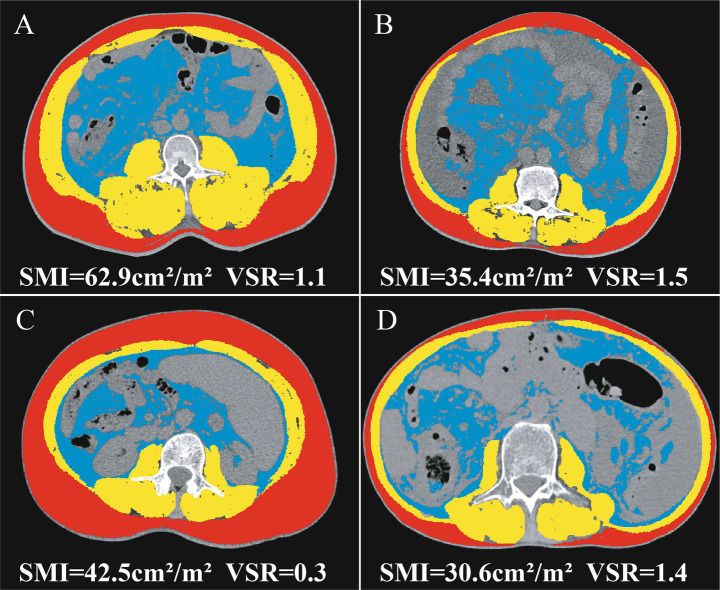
Computed tomography images at the L3 level used to assess low muscle mass and visceral adiposity (LMM-VA). (A) Male patient without LMM-VA; (B) Male patient with LMM-VA; (C) Female patient without LMM-VA; and (D) Female patient with LMM-VA. Yellow areas indicate skeletal muscle mass; red areas indicate subcutaneous adipose tissue; and blue areas indicate visceral adipose tissue.
